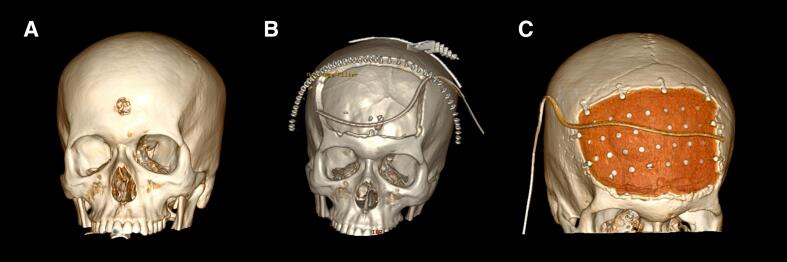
3D reconstructions of preoperative CT Brain (A), post decompressive bifrontal craniectomy CT Brain (B), post cranioplasty CT Brain (C).
